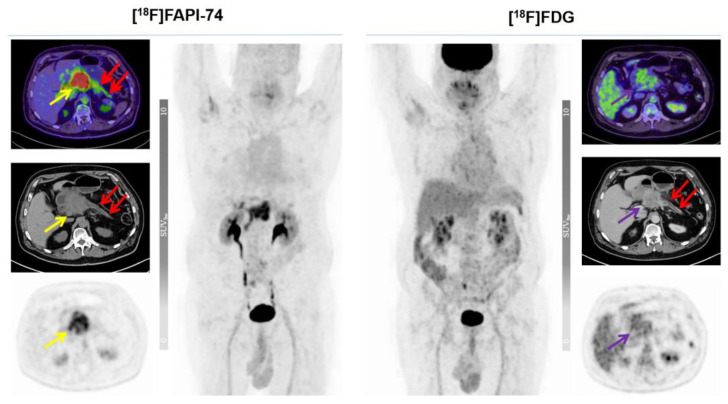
A 66-year-old male patient after palliative chemoradiotherapy with a confounding diffuse pancreatitis and remarkable [^18^F]FAPI-74 uptake in the primary lesion. Yellow and violet arrows indicate the primary lesion, while red arrows address the confounding pancreatitis of the rest of the pancreas (SUV_max_ of the primary lesion in [^18^F]FDG and [^18^F]FAPI-74; 3.4 vs. 10.7, respectively) as in the case depicted in Figure 5.
